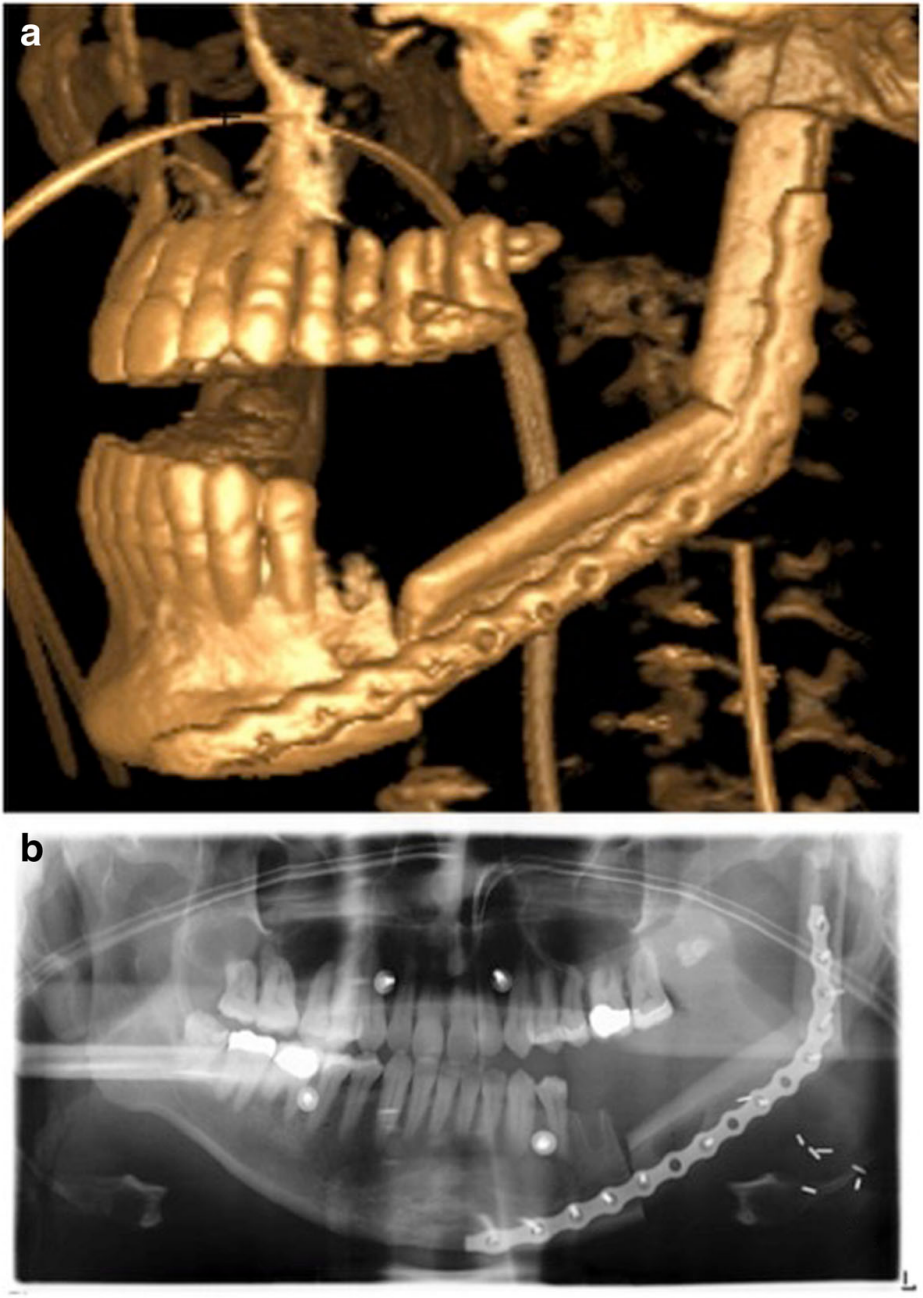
a b postoperative 3D reconstruction of the fibula graft (1 day after surgery) and panoramic x-ray (7 days after surgery)
